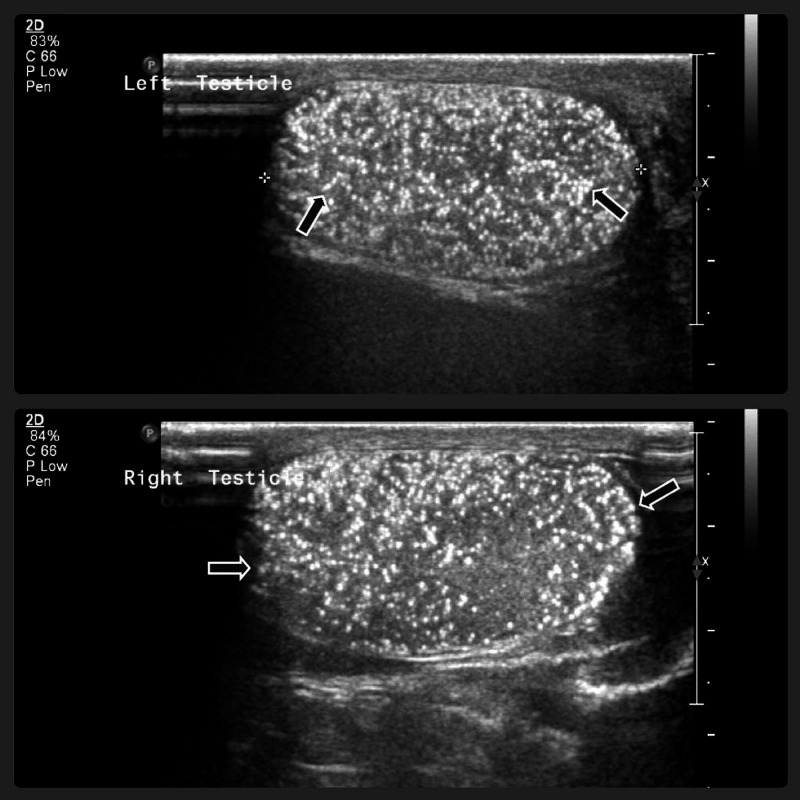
Sonographic appearance of the testes shows diffuse testicular calcifications consistent with microlithiasis.
